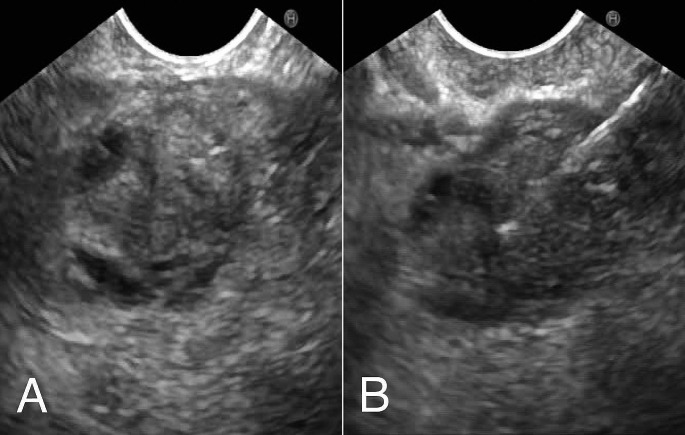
Endoscopic ultrasound images of a 15 mm solid lesion (A) detected in the pancreatic head of a 54-year old patient with Peutz-Jeghers syndrome. The lesion had low uptake of contrast (not shown). On MRI/MRCP the lesion was dubiously present and could not be characterized. On CT it was not visible. At revaluation with endoscopic ultrasound two months later, the lesion was unchanged and fine-needle aspiration (B) was suggestive of malignancy. The lesion was resected and staged as a T1aN0M0 pancreatic ductal adenocarcinoma
